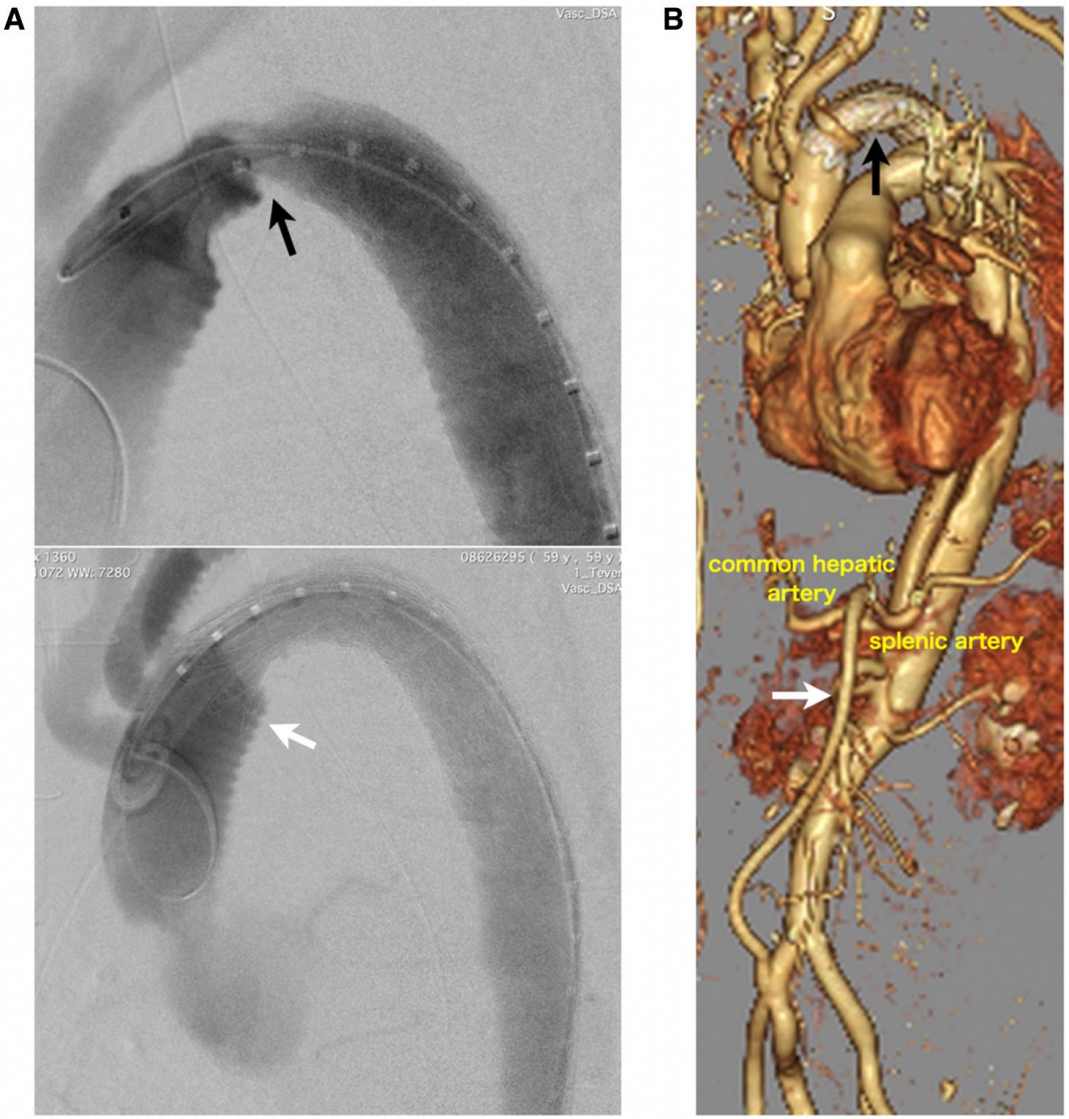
(A) Angiography conducted during thoracic endovascular aortic repair (TEVAR) indicates kinking of Frozenix graft at the junction (black arrow). The stent graft is located from the non-stented part (white arrow). (B) Post-TEVAR CT angiography shows that the kink was corrected (black arrow) and an ilio-coeliac artery bypass is placed (white arrow).
